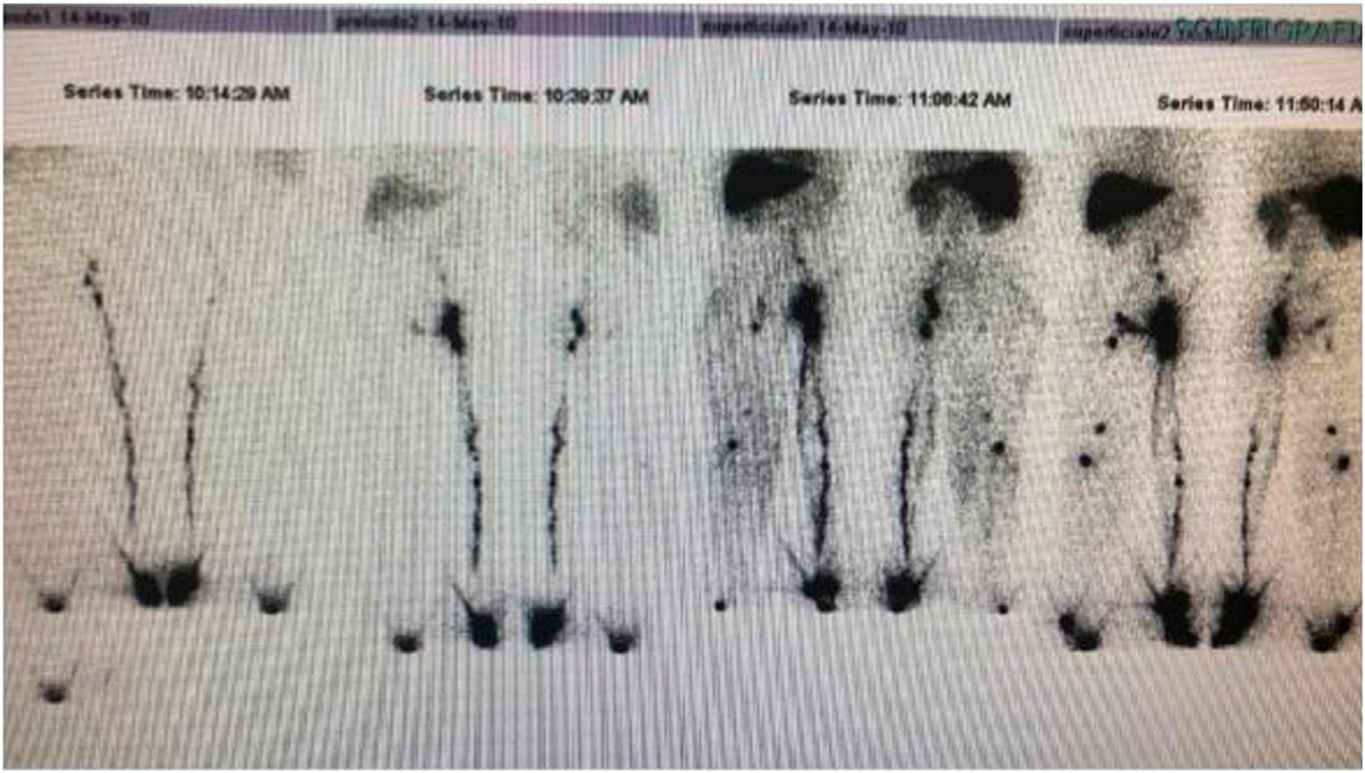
Lymphoscintigraphy: Lack of superficial and deep lymphatics in the right lower limb Lymphatic malformations are often associated to Klippel Trenaunay malformation.
